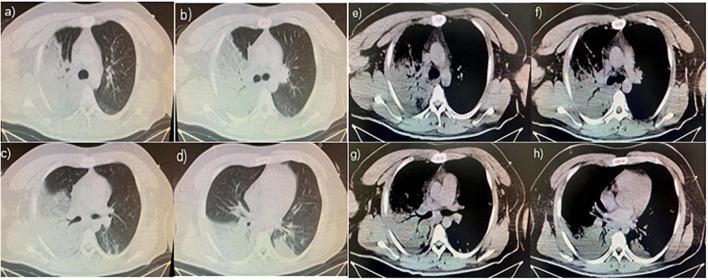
(a–h) The chest computer tomography (CT) scan displayed a double lung infection.
